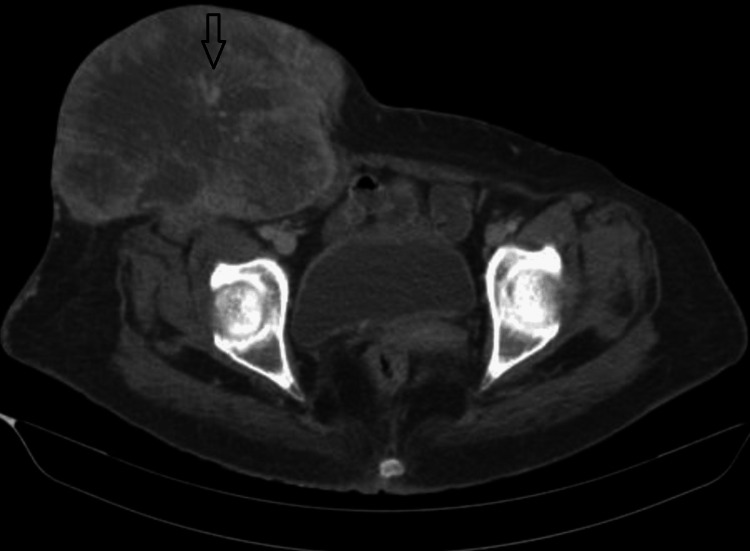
CT angiography showing the arterial supply of the tumor from the feeder vessel (black arrow) CT: computed tomography
